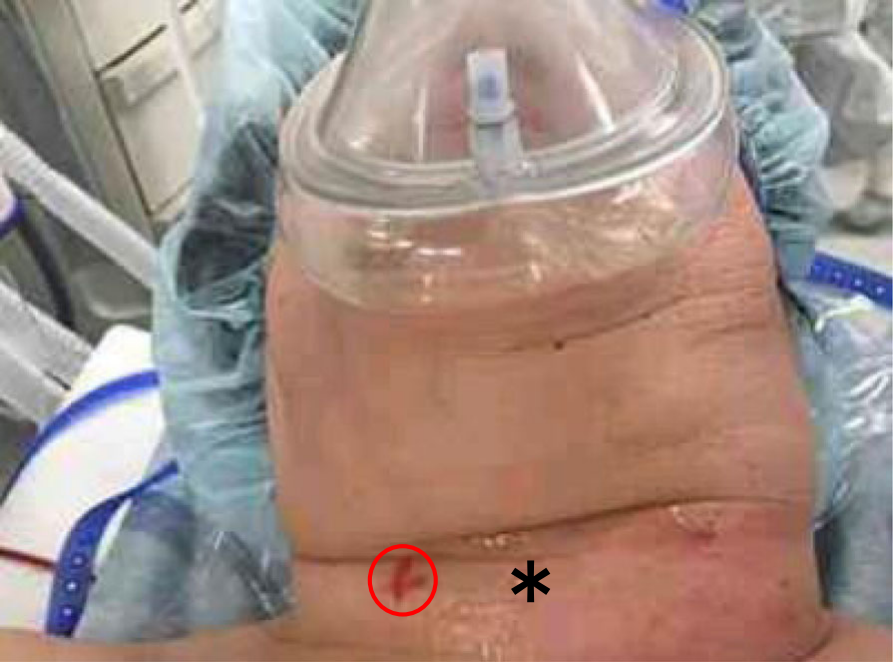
The patient’s neck The asterisk indicates the area palpated by the surgeon to find the cricothyroid membrane. The red circle shows the cricothyroid membrane identified by ultrasound
